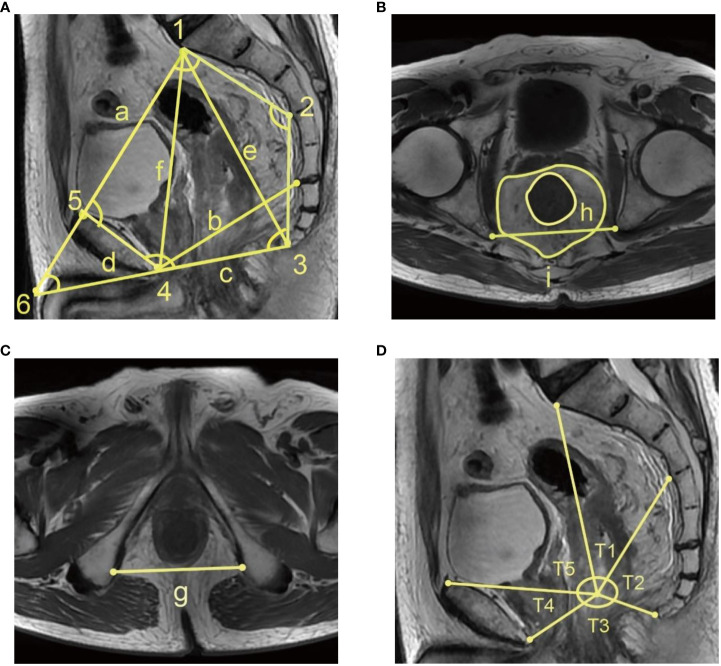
MRI T2 weighted image pelvimetry (A) Median sagittal position: (a) pelvic inlet, (b) middle pelvis, (c) pelvic outlet (d) Pubic symphysis height, (e) sacrococcygeal distance, (f) Internal diameter of sacrum and pubis (1), Angle 1 (2), Angle 2 (3), Angle 3 (4), Angle 4 (5), Angle 5 (6), Sacrococcygeal–pubic angle (B) Ischiatic tuberosity horizontal transverse position:(g) Intertuberous distance (C) Fifth sacral vertebral tip horizontal transverse position: (h) mesorectal fat area, (i) Interischial distance (D) Median sagittal position: (T1) Angle T1, (T2) Angle T2, (T3) Angle T3, (T3) Angle T3, (T4) Angle T4, (T5) Angle T5.
